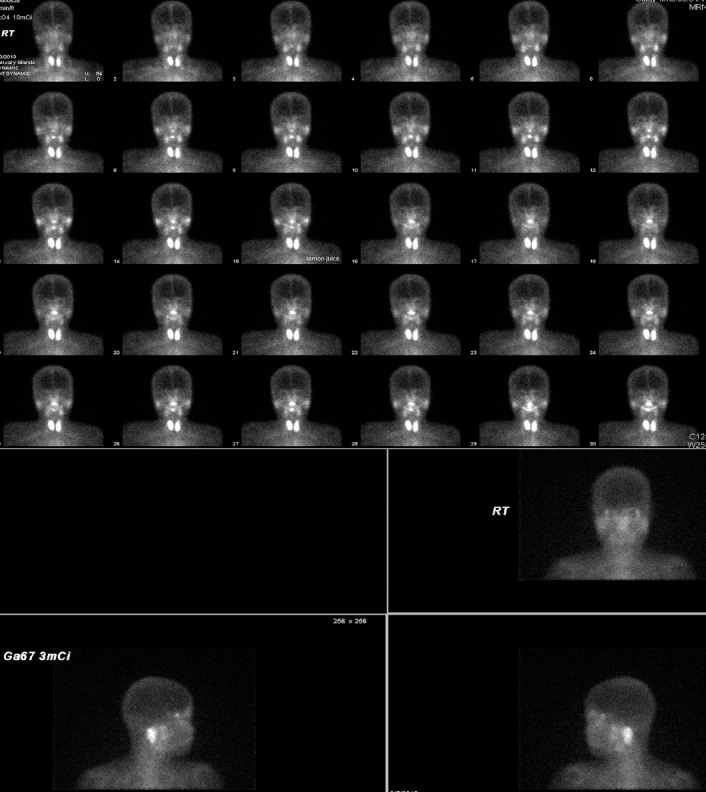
Images of a 43-year-old woman (Patient 4). (A) ^99m^Tc-pertechnetate scintigraphy exhibits normal accumulation of tracer in both parotid and submandibular glands with normal excretory function after stimulation (lemon juice at 15 min). (B) Gallium scan shows moderate to marked increased uptake in the bilateral parotid glands and mild increased uptake in the lacrimal glands
